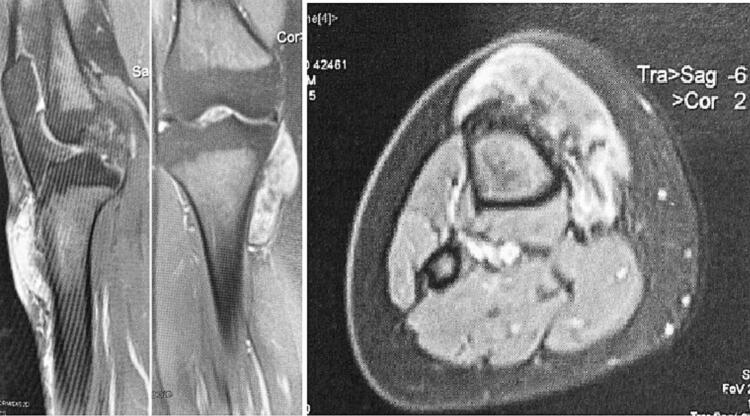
MRI images demonstrating invasion of the anterior tibial tuberosity and the patellar tendon without extension to the posterior and external soft tissues of the leg.
